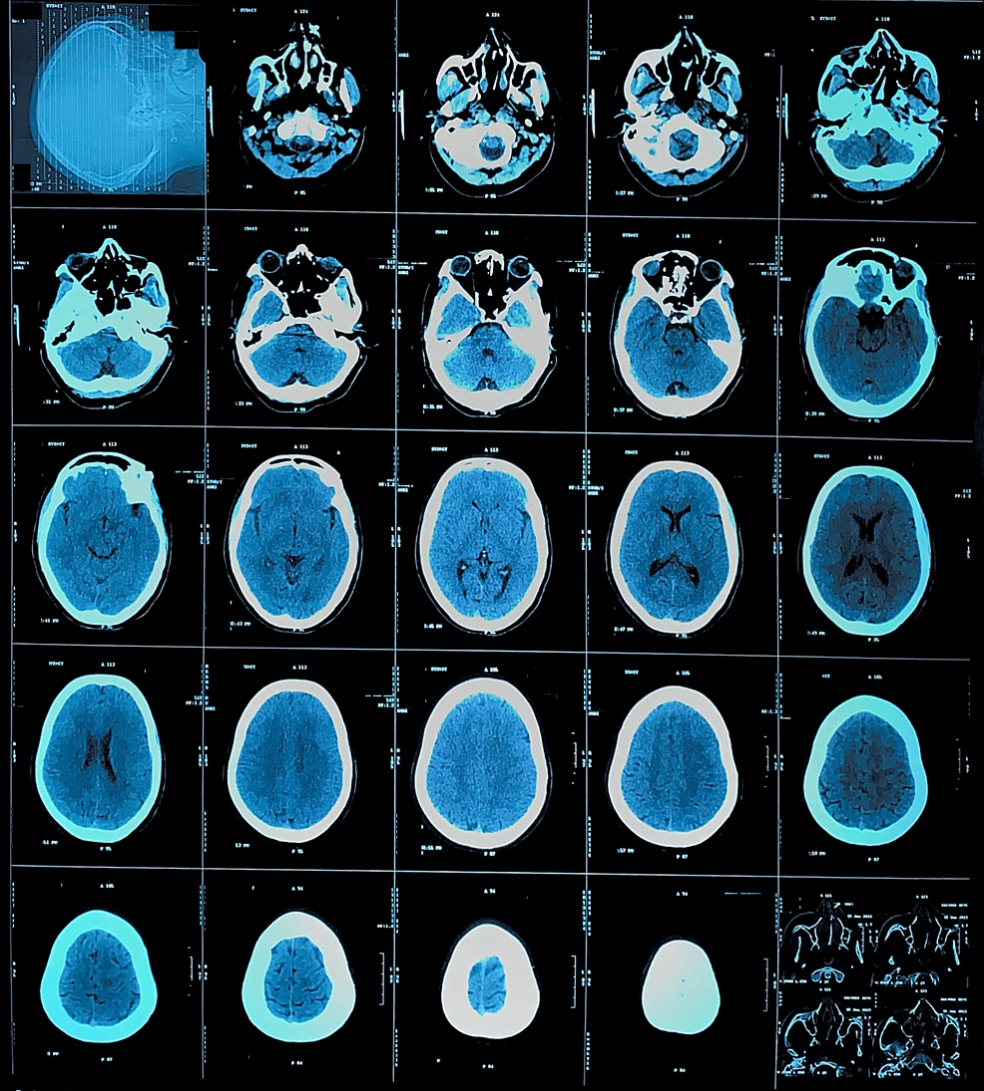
Plain Axial View CT Scan of Head
